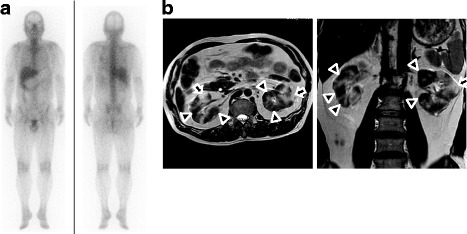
Findings of Ga-67 scintigraphy and MR imaging. (a) No uptake to kidney by Ga-67 scintigraphy and (b) low-intensity signals (*arrow head*) and high-intensity signals (*arrow*) by T2-weighted MR imaging
